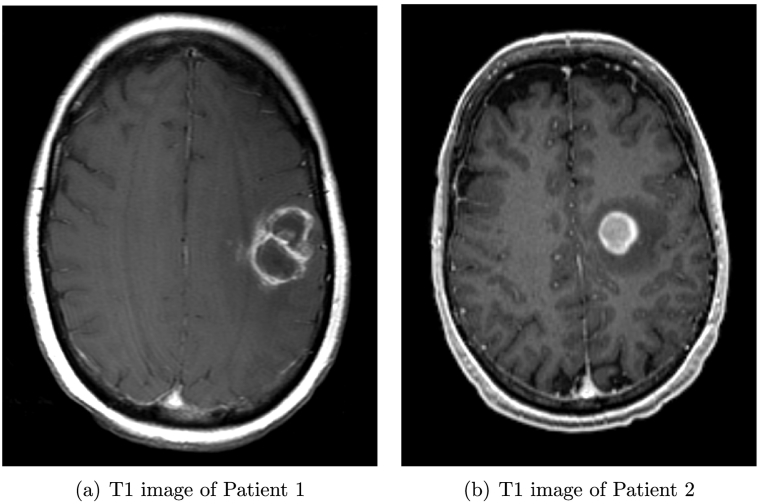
(a) Axial image from Patient 1 with a left fronto-parietal glioblastoma multiforme tumor and peritumoral edematous zone. (b) Axial image from Patient 2, with two metastatic lesions of unclassified pleomorphic sarcoma in the left frontal lobe and peritumoral edema. Gadolinium was given before the MRI scans.
